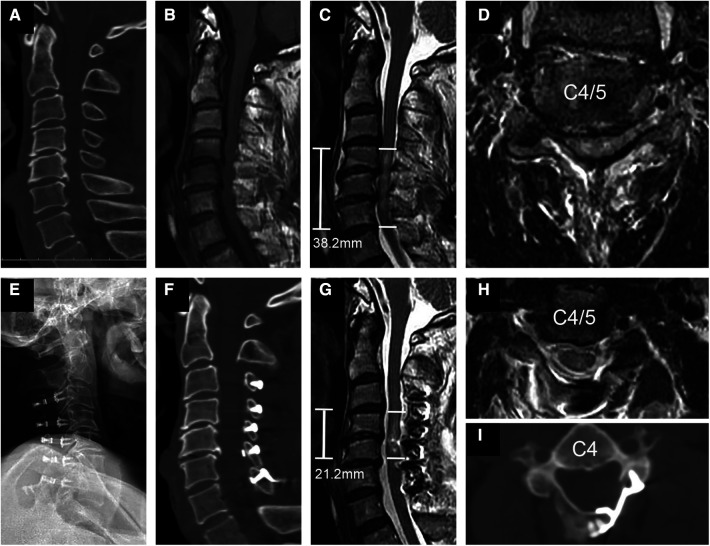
Representational preoperative (A–D) and postoperative (E–I) images of a 58-year-old male patient who sustained a spinal cord injury without fracture or dislocation. His AMS was 18 and AIS grade C. Midsagittal subaxial CT (A) indicated cervical spinal stenosis (CSS) from C3 to C6, and MRI (B) SCI with an intramedullary lesion length (IMLL) of 38.2 mm. The subarachnoid space (SAS) was absent at C4 and C5 (C,D). Laminoplasty of C3–7 was performed in this patient 17 h after trauma. Postoperative x-rays (E) and CT (F,I) showed significant enlargement of the osseous spinal canal. Postoperative MRI showed an IMLL of 21.2 mm (G) and successful decompression (G,H) indicated by the presence of an open anterior and posterior SAS 11 days after trauma. One year after injury, the patient recovered from paralysis with an AMS of 100 and AIS grade of E.
